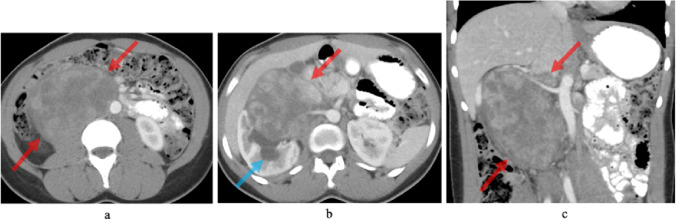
Axial (a, b) and coronal (c) contrast-enhanced CT images show a large heterogeneously enhancing right retroperitoneal mass (red arrows). The lesion has low density components and exerts mass effect on the right kidney with hydronephrosis (blue arrow). The patient had biopsy proven leiomyosarcoma arising from the IVC
